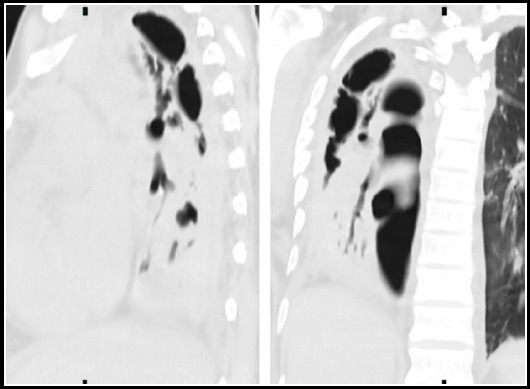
CT chest in lung window settings shows right sided pneumothorax which appears to be communicating with right sided bronchi consistent with bronchopleural fistula.
